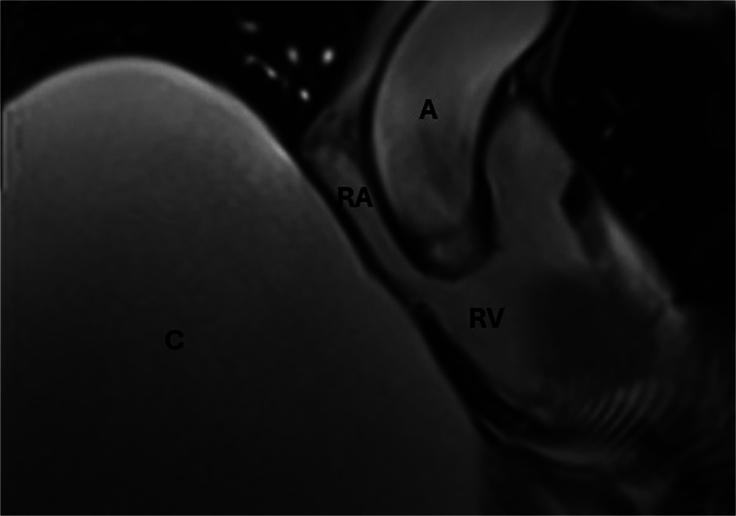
Cardiac MRI reveals the compression of right atrium (RA) and right ventricle (RV) due to a massive hepatic cyst (C). A = aorta; MRI = magnetic resonance imaging.
